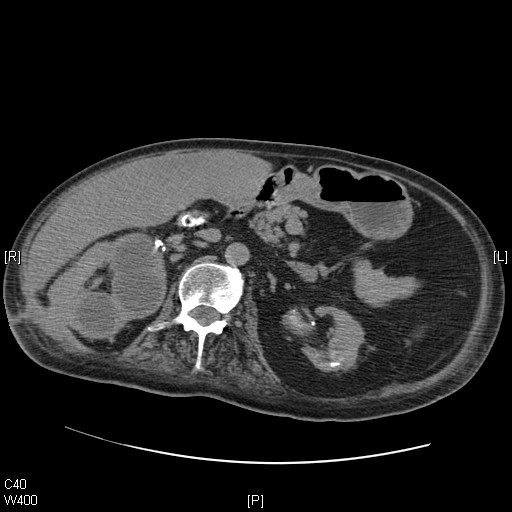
Axial CT scan of the abdomen, performed on 5 March 2007. Cysts in upper pole of right kidney are shown, the largest measuring 7 cm. In contrast to Figure 1, there was a marked loss of fat plane between the mid-pole of the right kidney and the postero-lateral abdominal wall.
